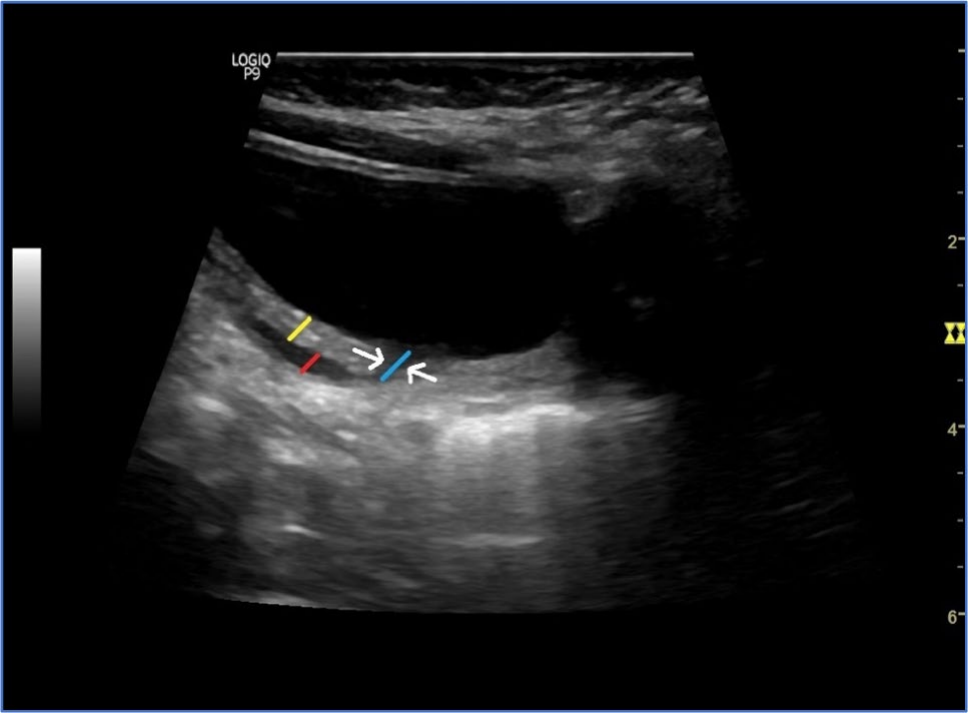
Ultrasonographic measurement. Yellow line: bladder wall thickness. Red line: distal ureteral diameter. Blue line: detrusor-ureteral orifice distance; the white arrow indicates the ureter inside the detrusor
